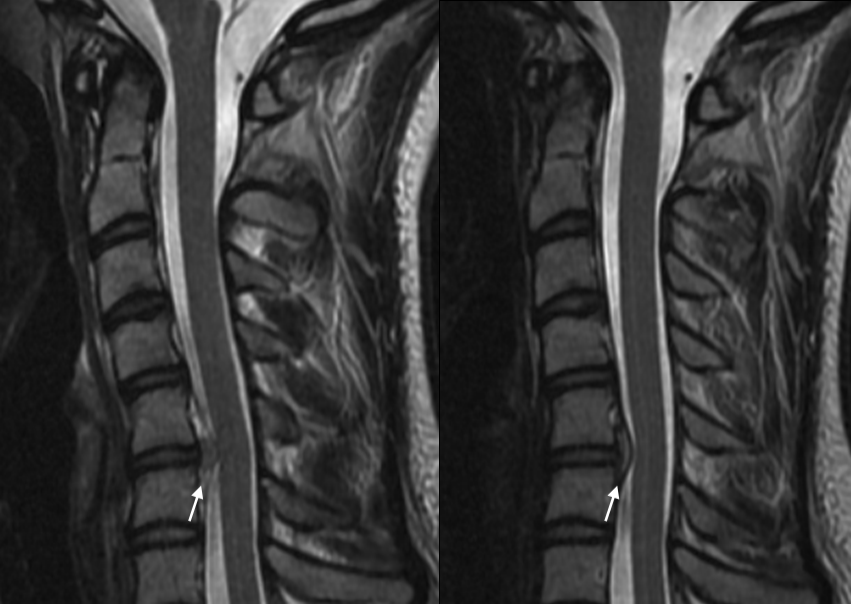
Sagittal magnetic resonance imaging (T2). The magnetic resonance imaging scan of the cervical spine on the day of trauma (A) shows an epidural mass (arrows) dorsally to C5/C6 - probably a hematoma or a disc protrusion, without signs of myelopathy. Six weeks later (B) the mass has decreased in size, the remaining disc C5/C6 is intact.
